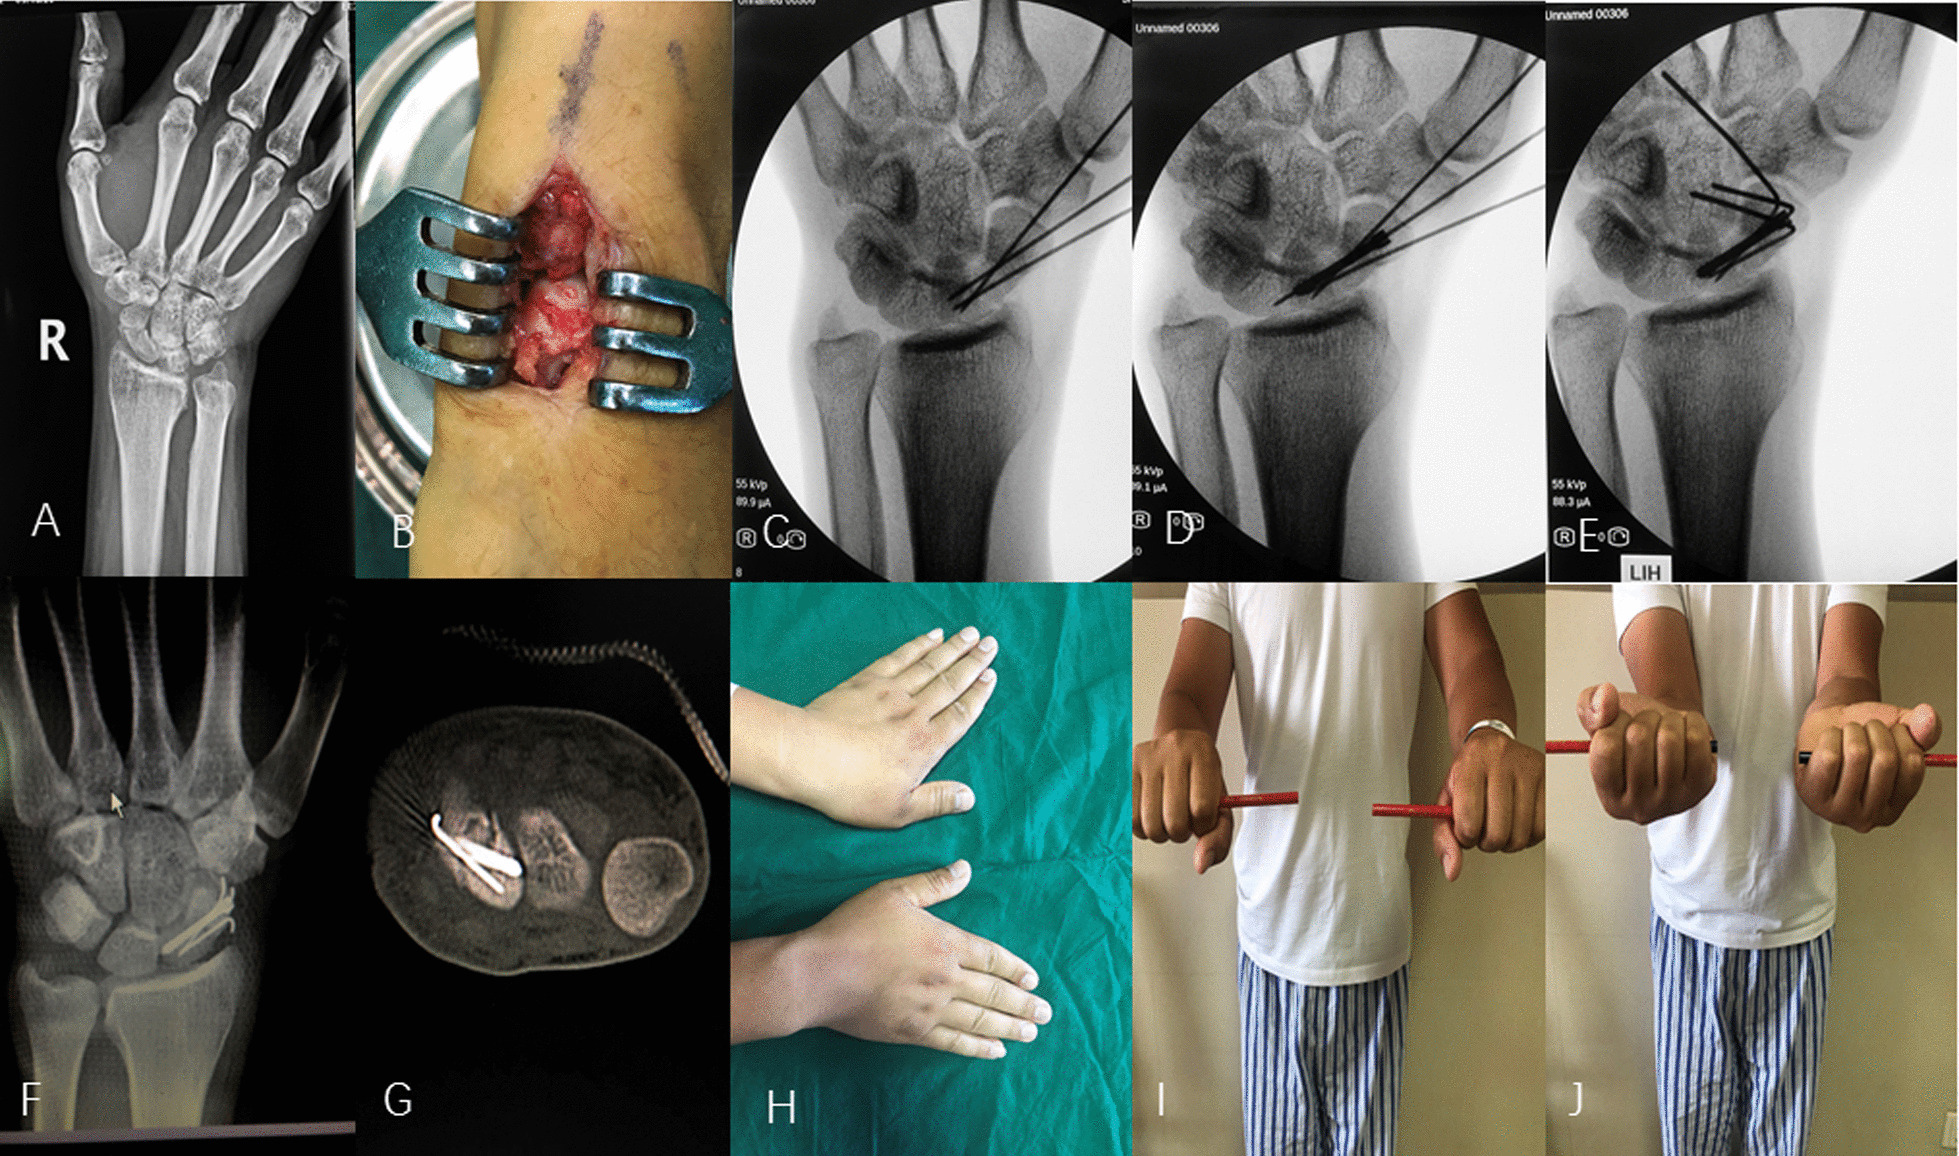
A 32-year young man sustained right scaphoid waist fracture nonunion 11 months after the initial fracture treated by conservative method. The a showed the nonunion at the scaphoid waist site. The b to e showed the operative process of tripod fixation using Herbert screw and two adjunctive K-wires. The f, g were the postoperative radiograph and the CT scan. h to j showed the excellent functional outcome at 12 months postoperatively
